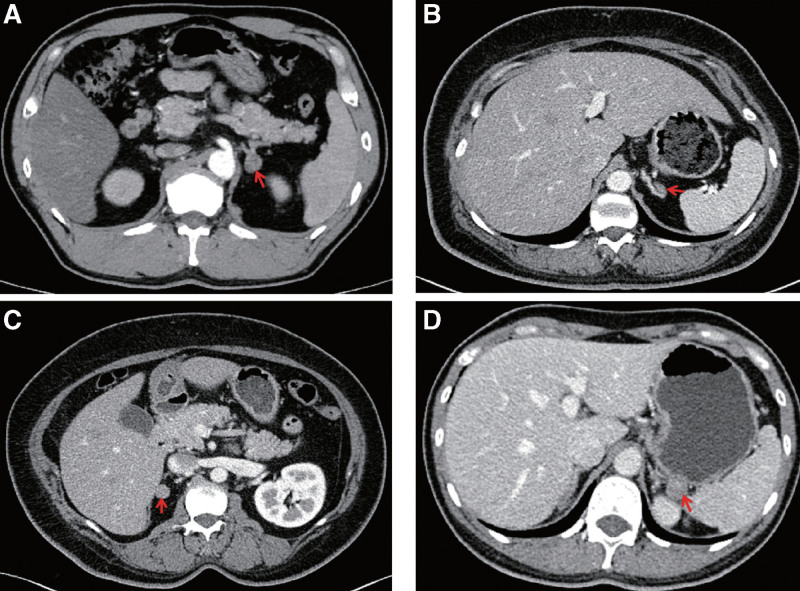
Results of adrenal contrast-enhanced CT of the 3 patients: (A) adrenal computed tomography enhancement in patient 1 indicating mild to moderate enhancement of left adrenal adenoma (pointed by the red arrow). (B) and (C) adrenal computed tomography enhancement in patient 2 shows heterogeneous enhancement of bilateral adrenal nodules (pointed by red arrows). (D) adrenal computed tomography enhancement in patient 3 indicating marked persistent enhancement of the left adrenal adenoma (pointed by the red arrow).
